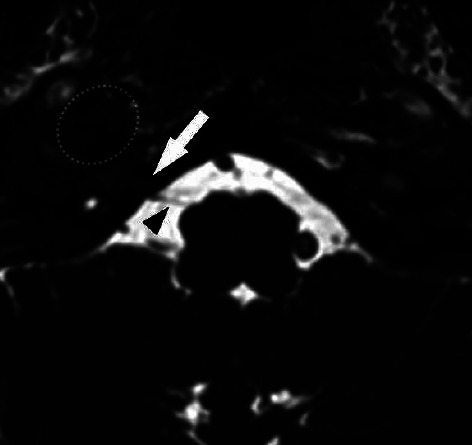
Axial steady-state free procession MR image through the skull base shows the hypoglossal nerve (arrowhead) passing through the hypoglossal canal (arrow). The osteophyte (dashed oval) lies just anterior to the hypoglossal canal, along the expected path of the hypoglossal nerve.
